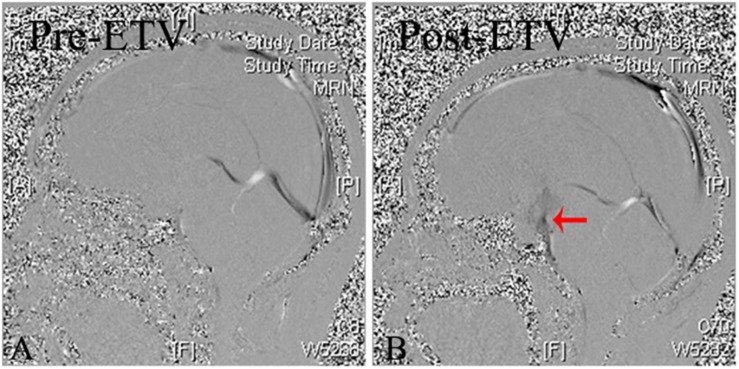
Cine-MRI of pre- and post-ETV. (A) Pre-ETV sagittal Cine-MRI demonstrating no CSF flow at aqueduct and the outlet of the fourth ventricle. (B) Post-ETV sagittal Cine-MRI demonstrating the moving CSF flow across the bottom of the third ventricle (arrow).
